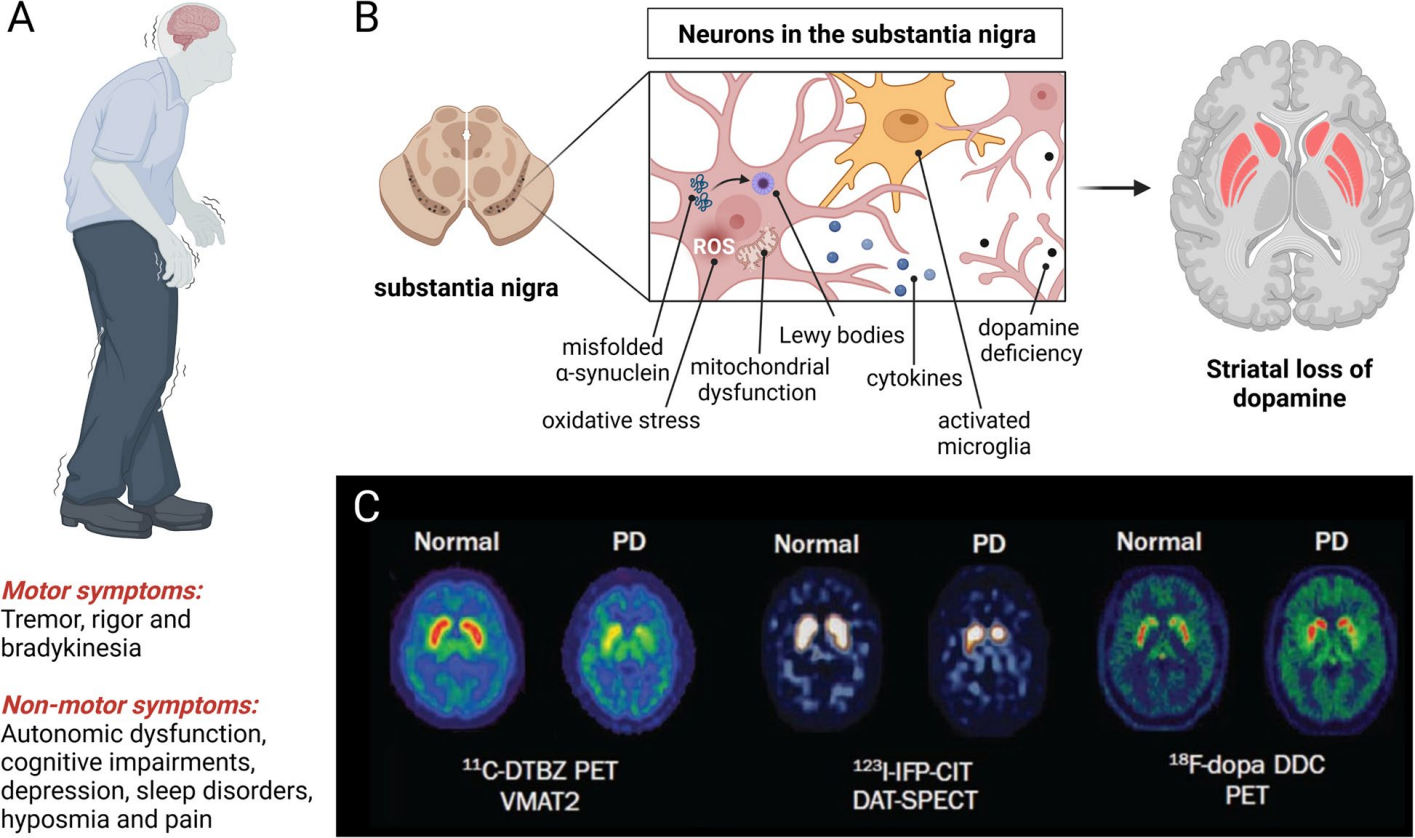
Clinical presentation, pathophysiology and diagnostic imaging in Parkinson’s disease (PD). A Cardinal motor symptoms and non-motor features of PD. B Pathological hallmarks of PD include aggregates of misfolded α-synuclein (Lewy bodies), mitochondrial dysfunction & oxidative stress, infiltration of immune cells and persistent activation of microglia, as well as an increased release of cytokines. The pathophysiology of PD prompts neurodegeneration and dopamine deficiency, which is particularly pronounced in the mammalian striatum. C Translational molecular imaging with positron emission tomography (PET) and single-photon emission computed tomography (SPECT), visualizing striatal degeneration in PD non-invasively. DAT, dopamine transporter.; DDC, DOPA decarboxylase; VMAT2, vesicular monoamine transporter 2. Figure 1C reprinted by permission from the Royal College of Physicians (RCP): Pagano et al., [17] copyright (2016)
